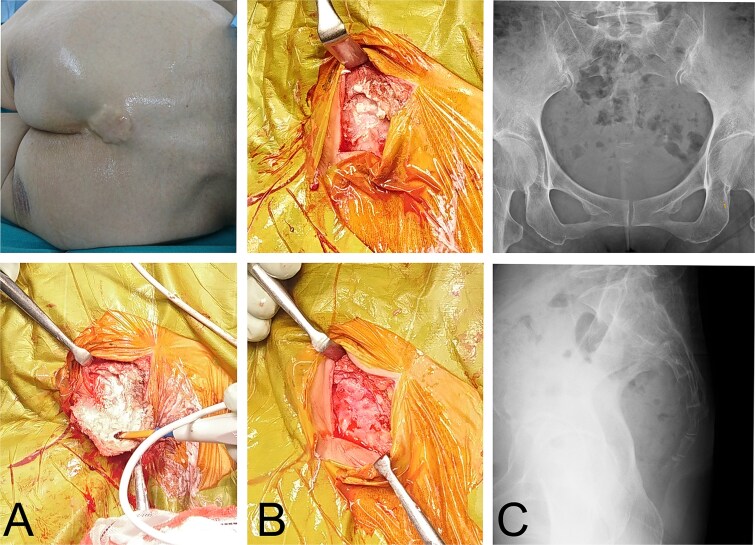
Clinical and intraoperative images. A sacral protuberance is visible (A). A white calcified lesion is exposed after skin incision (A). Residual calcified deposits on the fibrous wall are seen after resection (B) and decrease after irrigation with bicarbonate Ringer’s solution (B). Postoperative radiographs show no residual radiopaque material (C).
